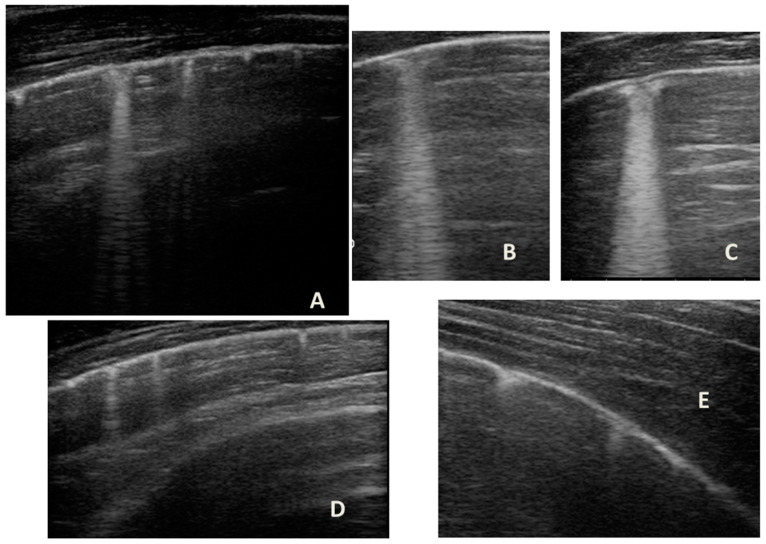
Grayscale lung ultrasound examination shows a picture of sonographic interstitial syndrome (SIS) represented by: (A): irregularity of the pleural line, short vertical artifacts, and long vertical artifacts unevenly distributed bilaterally; (B,C): irregularity of the pleural line, sub-centimeter consolidations associated with long confluent vertical artifacts located mainly in the right lung fields, mainly in the mid-apical area; (D,E): short vertical artifacts with pleural line irregularities unevenly distributed bilaterally.
